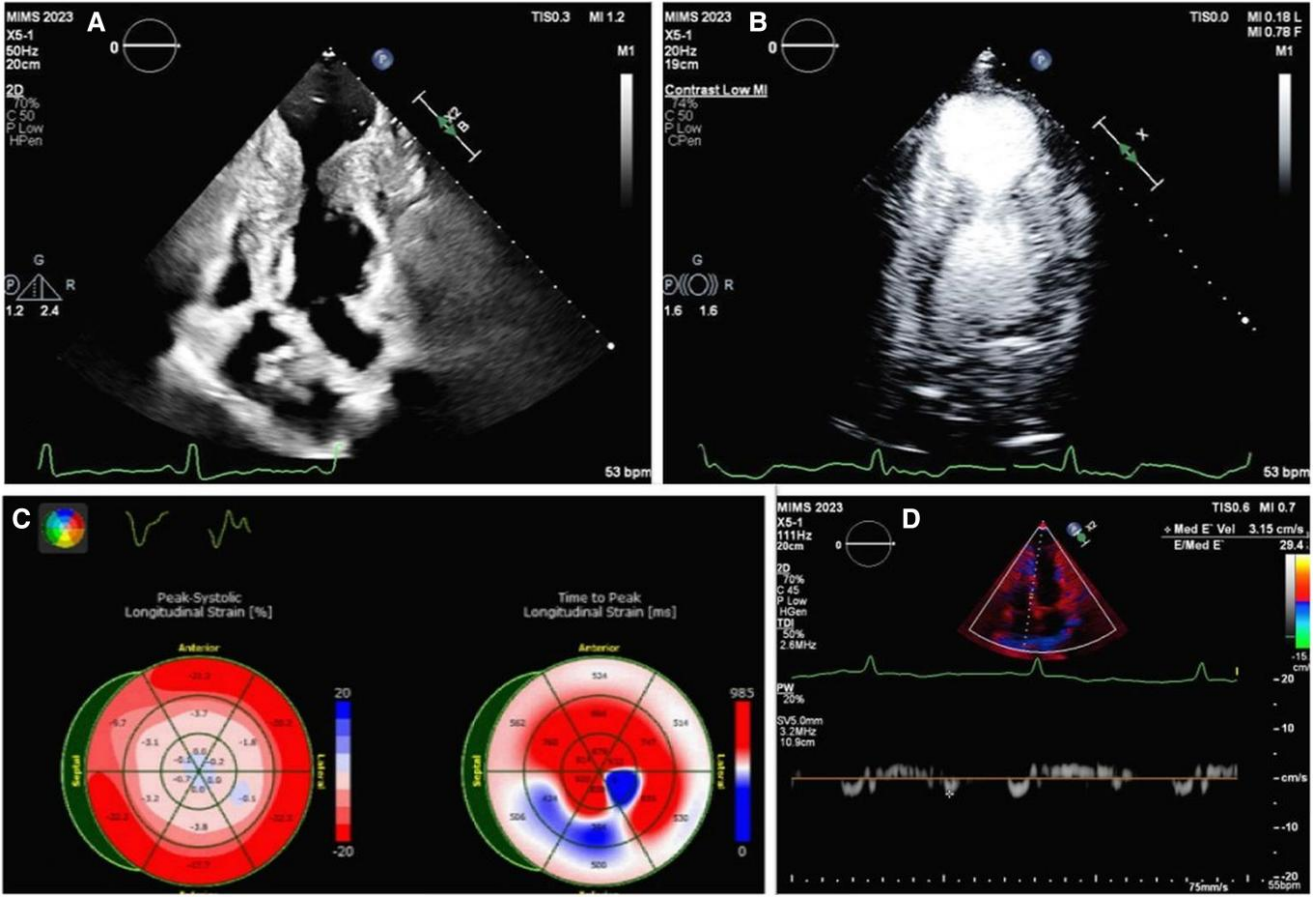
(A*) 2D echocardiogram in the apical four-chamber view showing the apical aneurysm and the apical and mid-septal left ventricular hypertrophy; *(B)* contrast echocardiography delineating the large apical aneurysm and confirming no thrombus; *(C)* global longitudinal strain analysis showing decreased apical strain values; *(D)* low mitral annular tissue Doppler velocity of 3.1 cm/s.
